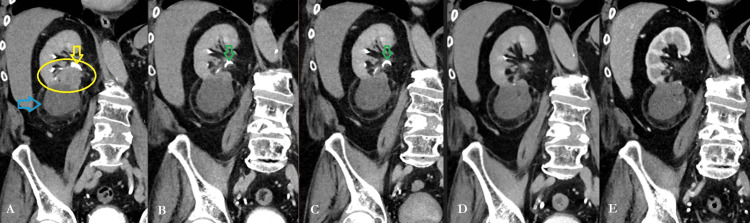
Representative pre- and posttreatment coronal CT images shown at the following intervals: (A) pre-SBRT, (B) four months post-SBRT, (C) six months post-SBRT, (D) 9.5 months post-SBRT, and (E) 15.5 months post-SBRT.
